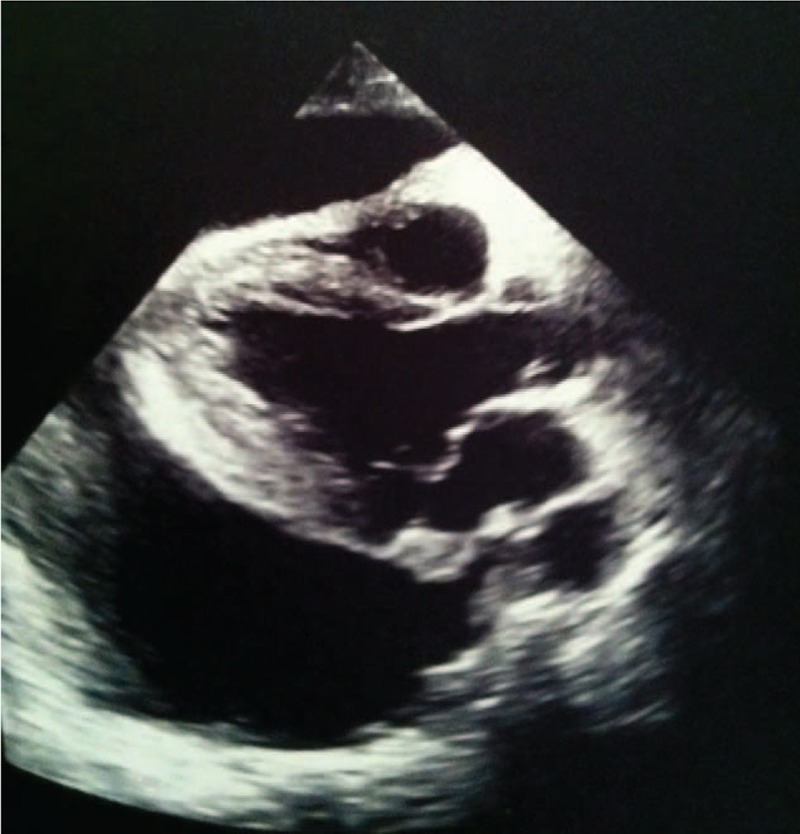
Transthoracic echocardiography: parasternal long axis view showing large pericardial effusion.
